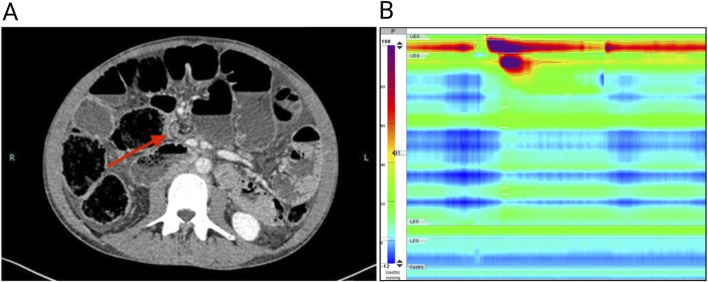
(A) Computed tomography of the abdomen showing a grossly dilated (6 cm diameter) small intestine; the arrow shows a jump in caliber close to the ileocecal valve. (B) High-resolution esophageal manometry shows the absence of esophageal contractility after wet swallows.
